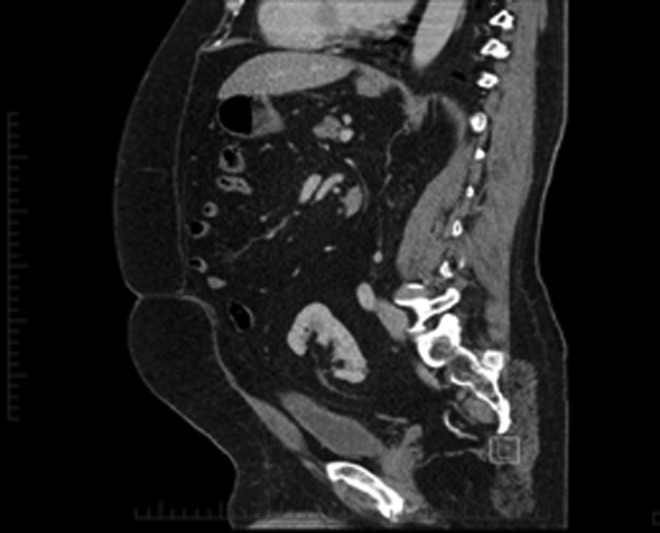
Case 1—CT urogram; sagittal image showing the proximity of ureter to the posterior aspect of the bladder in a congenital pelvic kidney.
